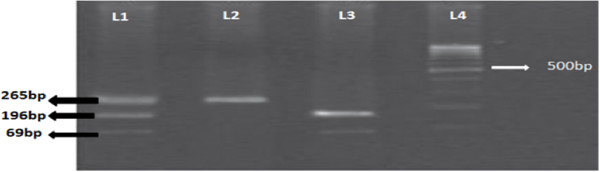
Fok I *Restriction digested PCR product of VDR gene. L4-100 bp molecular weight marker; L3-Homozygous TT genotype, L2-Homogygous CC genotype, L1-heterozygous CT genotype.
